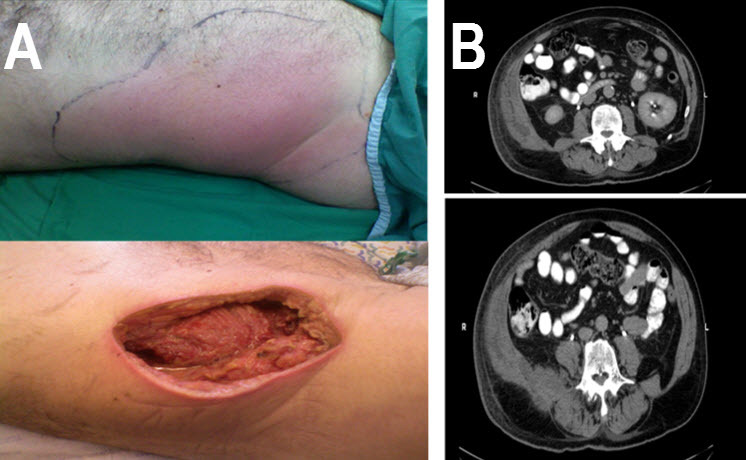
Photo and CT Scan of the Patient’s Right Flank. A: Right flank before and after surgery; B: CT scan of the afflicted area.
